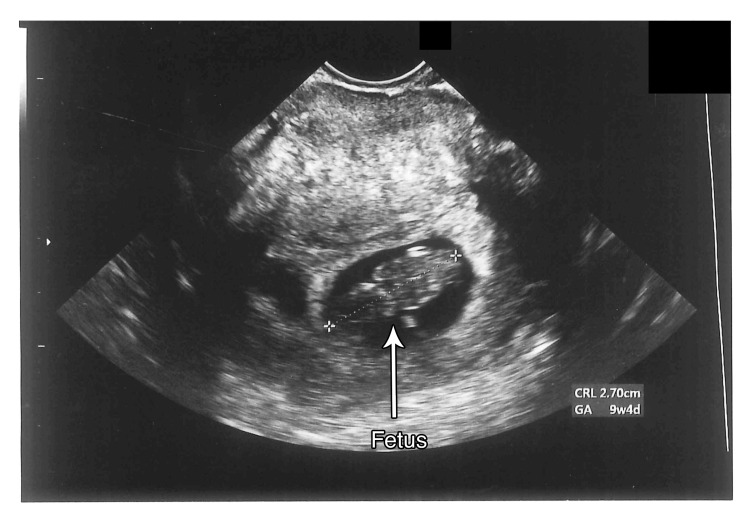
TVUS image of fetus (CRL 2.74 cm = 9 weeks and 4 days) TVUS: transvaginal ultrasound; CRL: crown-rump length
